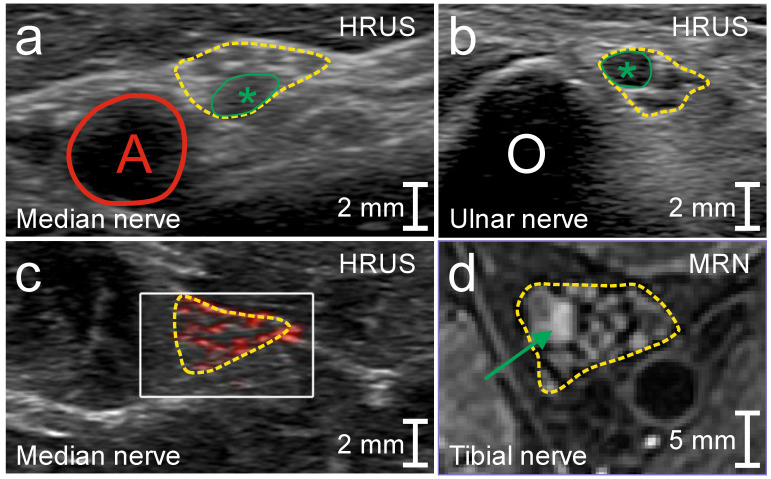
Peripheral nerve imaging in patient 1 (MMN). High-resolution nerve ultrasound (HRUS) demonstrated mild inhomogeneous enlargement of the cross-sectional nerve area (CSA, yellow in (a–d); (a) right upper arm median nerve, CSA 17 mm^2^; (b) left ulnar nerve at the cubital tunnel, CSA 13 mm^2^) and isolated prominent (green asterisk in (a), 3.7 mm^2^) or enlarged fascicle area (green asterisk in (b), 4.1 mm^2^) as well as increased nerve vascularizaton with multiple focal color-encoded spots (grade 2; within the yellow border in (c)). 7T magnetic resonance neurography (MRN) depicted isolated fascicles that were T2 hyperintense and enlarged (green arrow in (d); left tibial nerve). A, brachial artery; O, olecranon.
